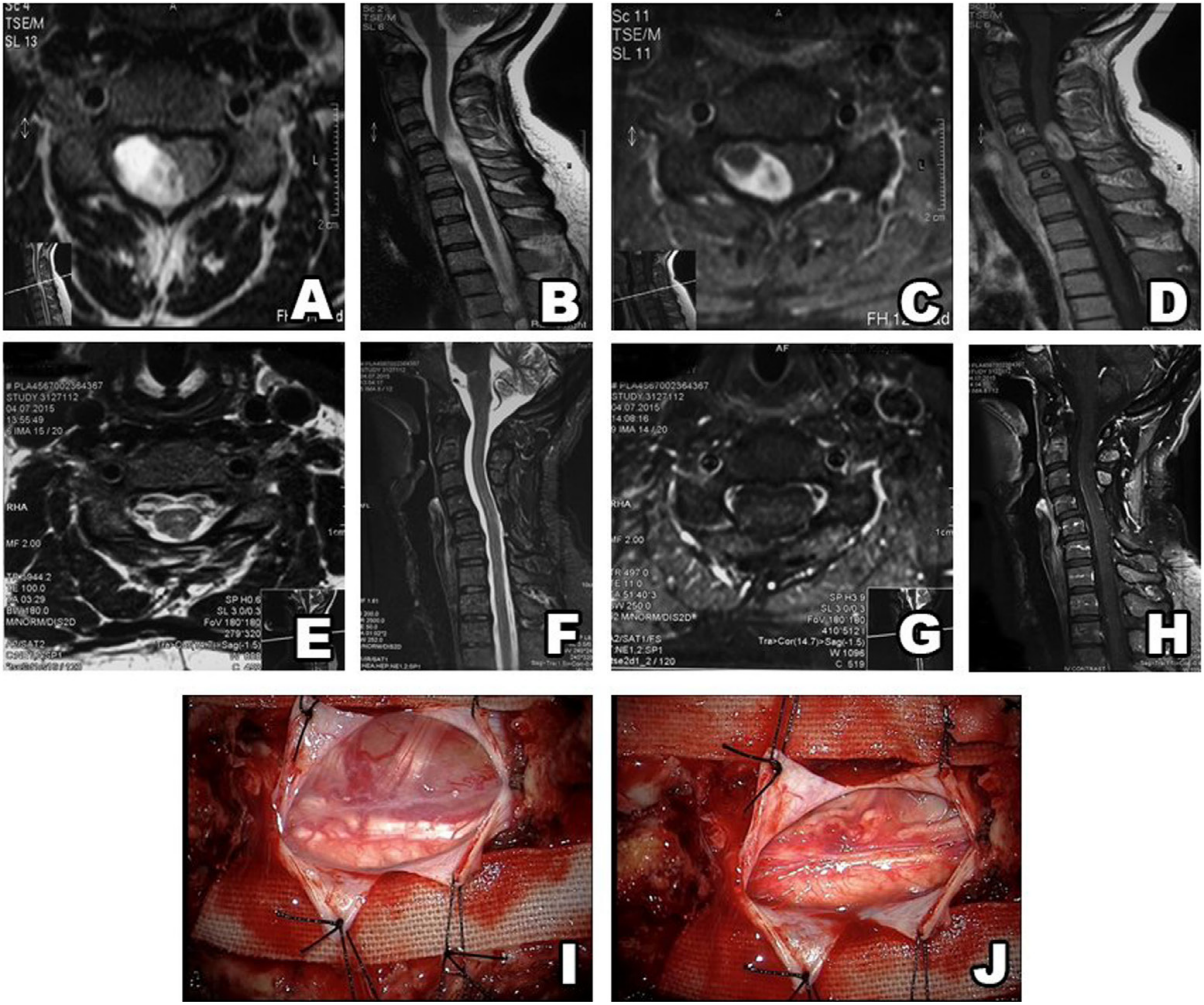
Type II schwannoma (intradural localization). MRI shows a heterogeneous, contrast-enhanced intradural tumor (A–D). Postsurgery MRI confirms gross total resection, without hematoma or recurrence (E–H). A schwannoma inside the dura (I). After resection, there is no residue remaining in surgical field (J).
